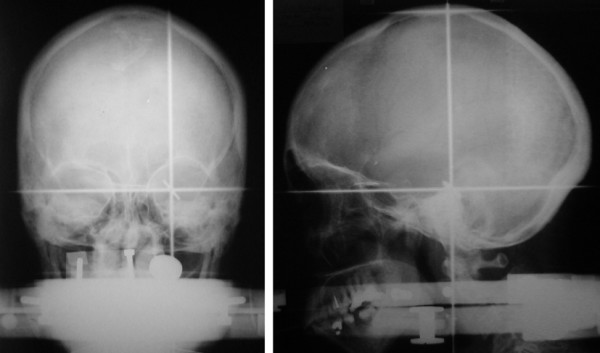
Orthogonal plain X-ray images; lateral and anterioposterior views.
